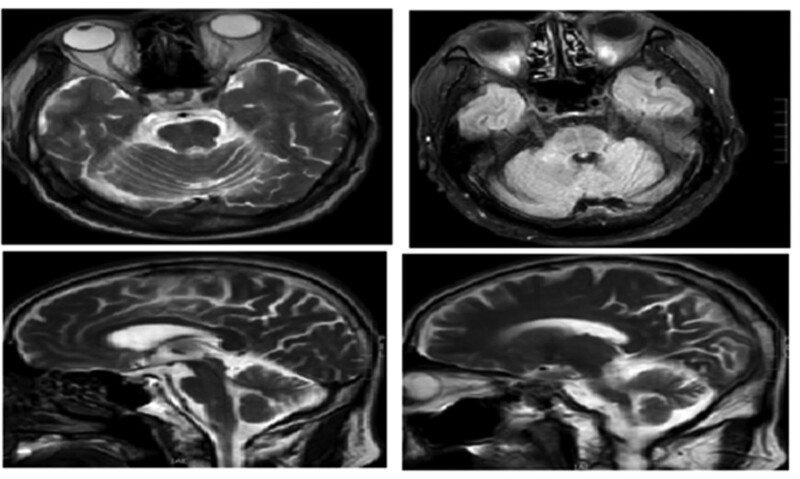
The cranial MRI did not show cerebellar and Brainstem atrophy. MRI = magnetic resonance imaging.
